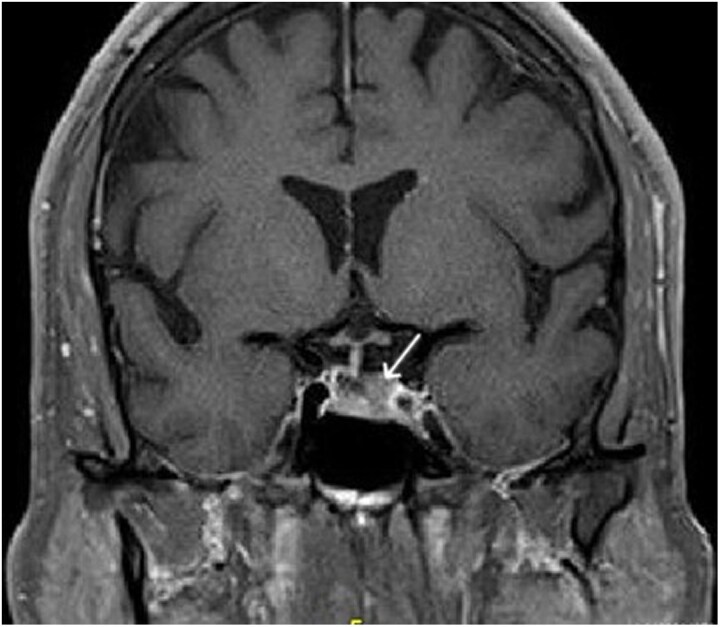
Pituitary magnetic resonance imaging before treatment with cabergoline for patient 1. Here, the left-sided pituitary macroadenoma is visualized, resulting in deviation of the gland and stalk to the right.
